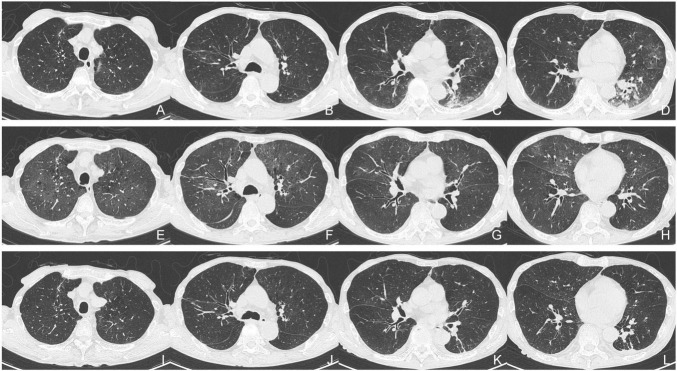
Serial chest computed tomography (CT). (A–D) Initial CT scan obtained during the patient’s first episode of COVID-19. (E–H) Second CT scan obtained on the patient’s readmission in August 2024. (I–L) Follow-up CT scan obtained before the patient’s discharge from hospital.
